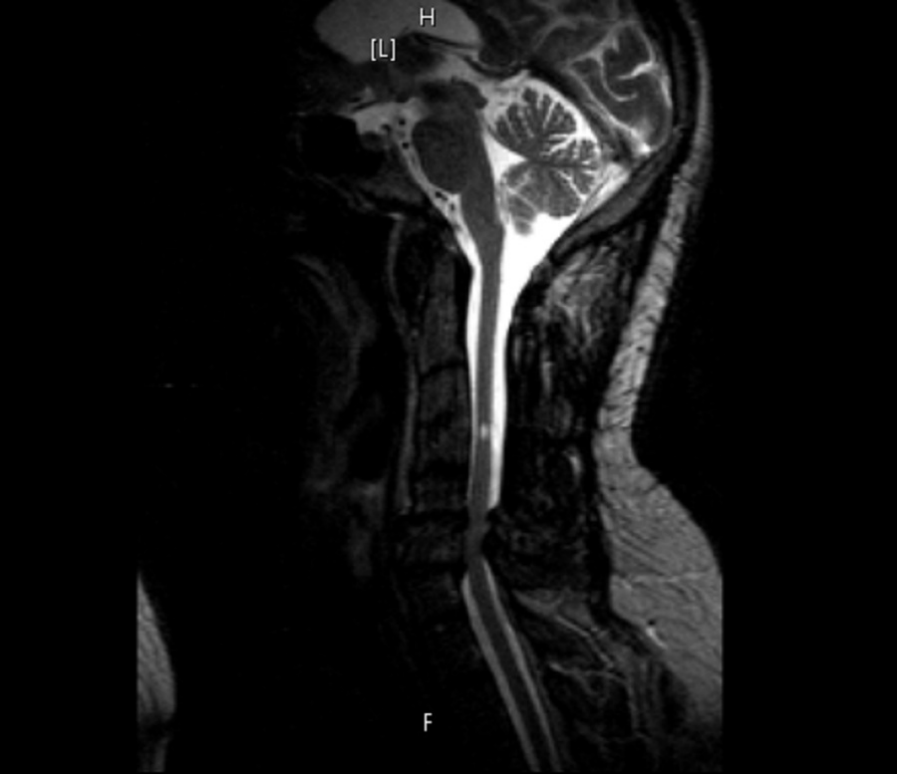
(Case 1) Preoperative sagittal MRI of cervical spine
